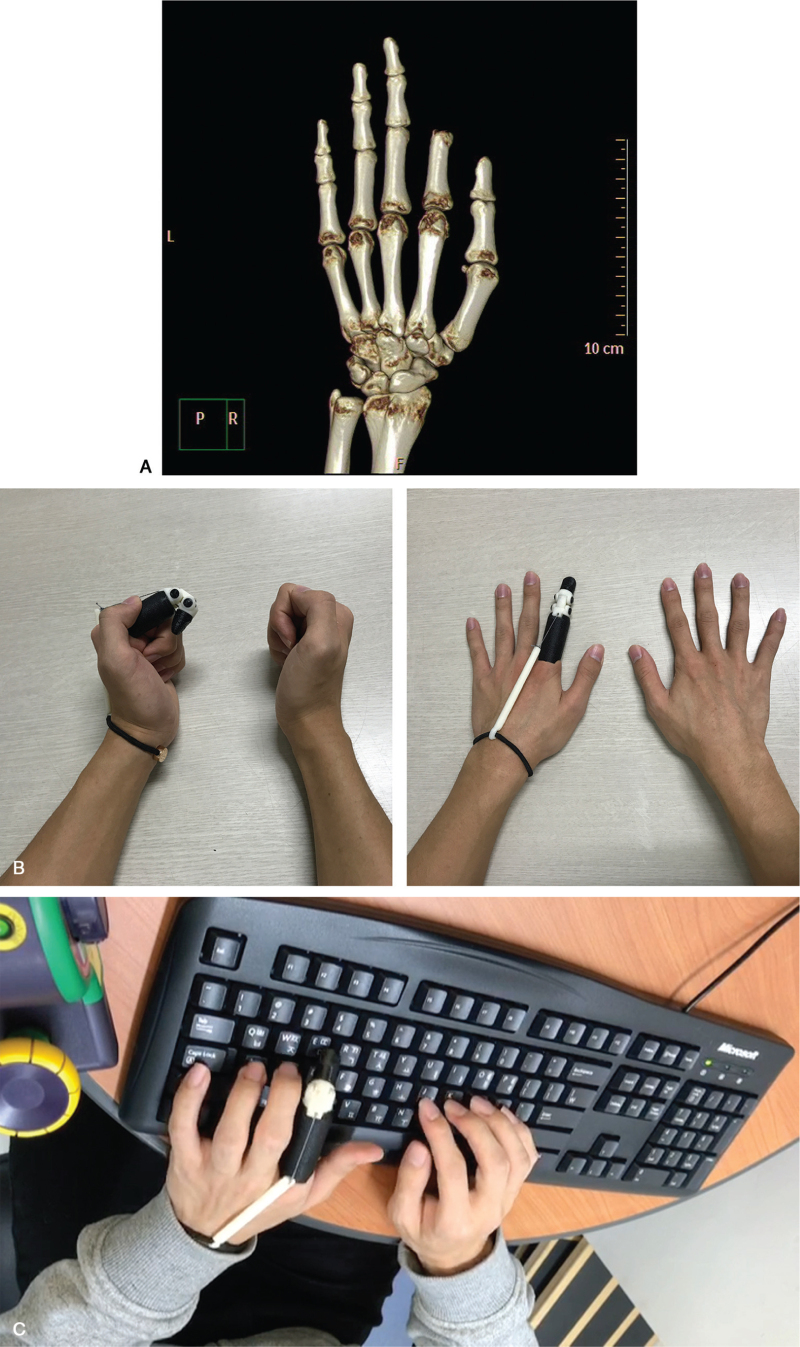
The case of patient 2. (A) A 3D-reconstructed bone structure from a computed tomographic scan of the left hand in patient 2. The amputation levels correspond to the second proximal phalanx. (B) The patient is wearing a body-powered 3D-printed finger prosthesis. (C) The patient is typing on an electronic keyboard.
